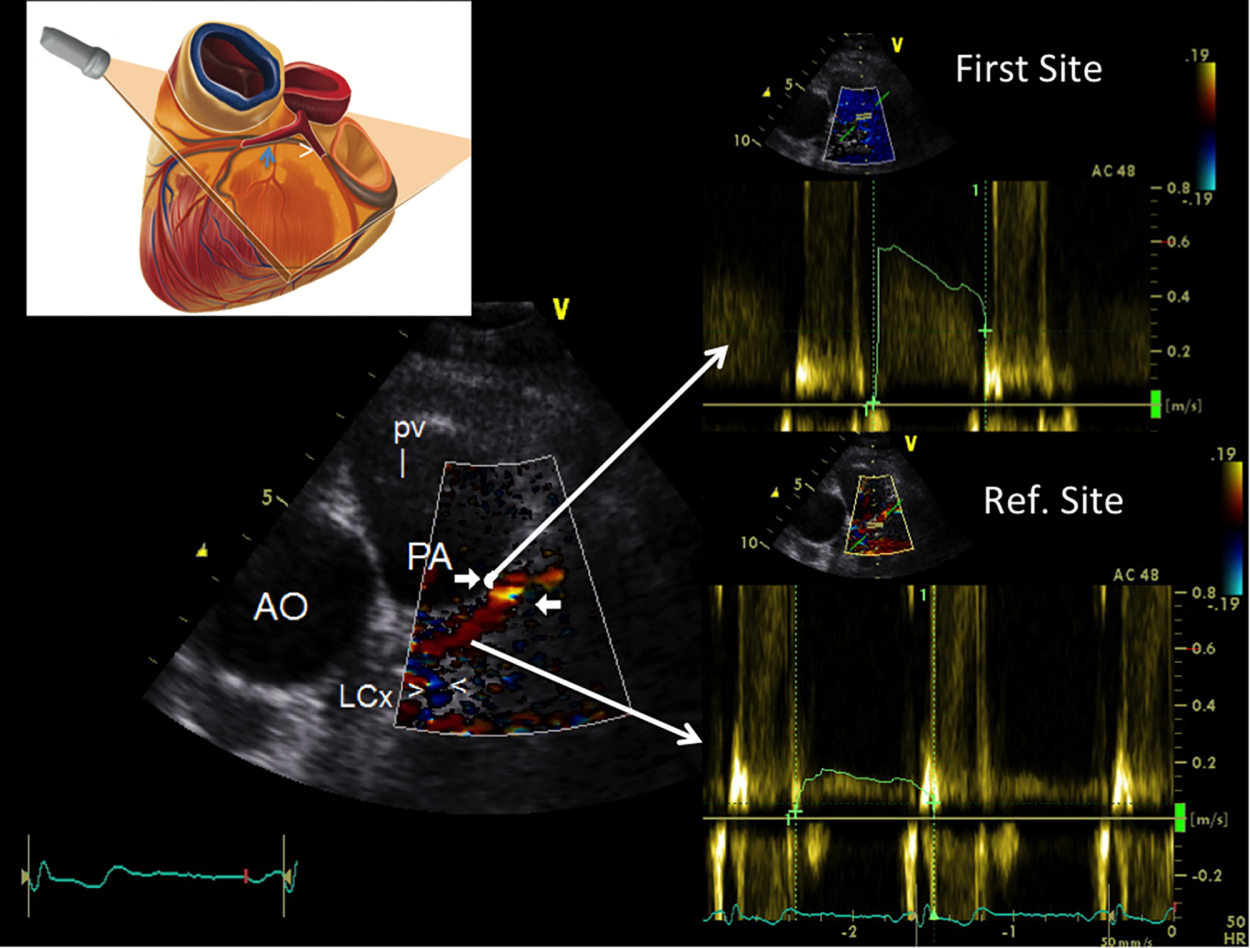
Example of how to perform color-guided PW Doppler sampling in the LAD. Plane orientation is shown on the top left. Color flow is recorded in the proximal LAD (coded in red) along with flow in the proximal LCx [blu signal as it is away from the transducer (arrowheads)]; an area of aliasing (accelerated flow) is shown in the proximal LAD (indicated by arrows); based on color guidance, PW wave Doppler recording is attained at the aliasing area first and then in a more proximal reference portion (reference site); the Doppler curves (on the right side) are then used to calculate %AsF and % CSA reduction. PW, pulsed wave Doppler; LAD, left anterior coronary artery; LCx, left circumflex coronary artery; AsF, accelerated stenotic flow; CSA, cross sectional area.
